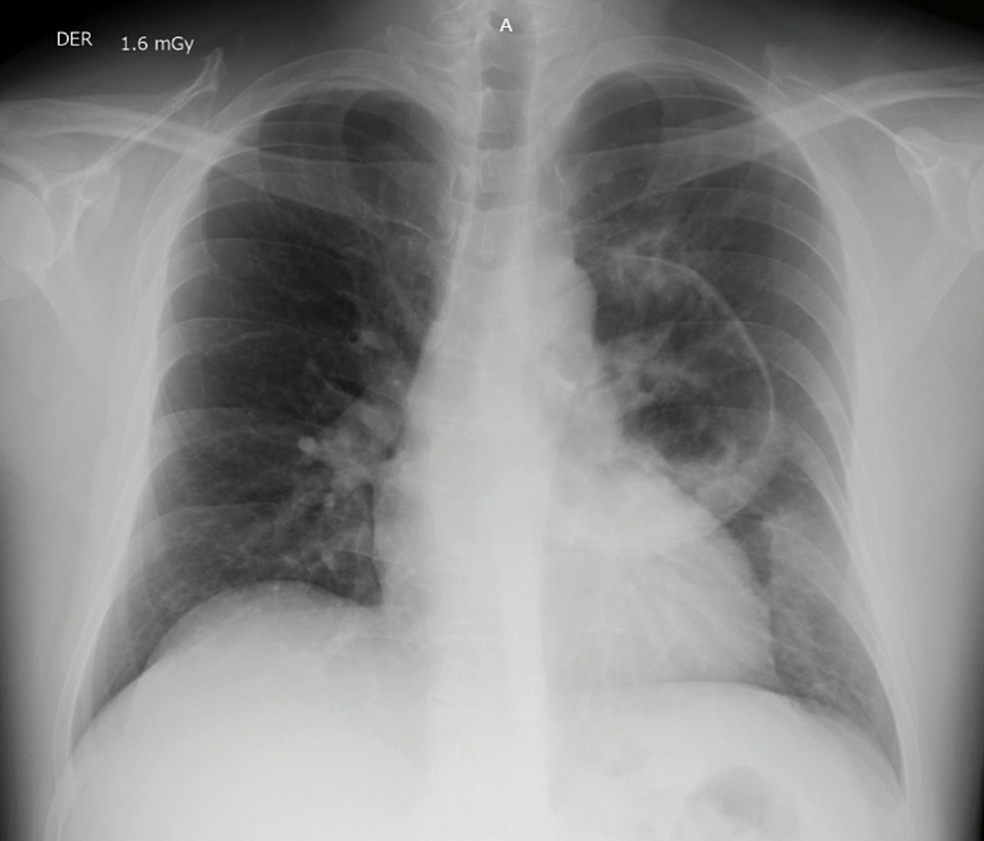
Anteroposterior chest X-ray. An anteroposterior chest X-ray was obtained in the emergency department upon initial presentation. A large left parahilar cavitation was observed.
